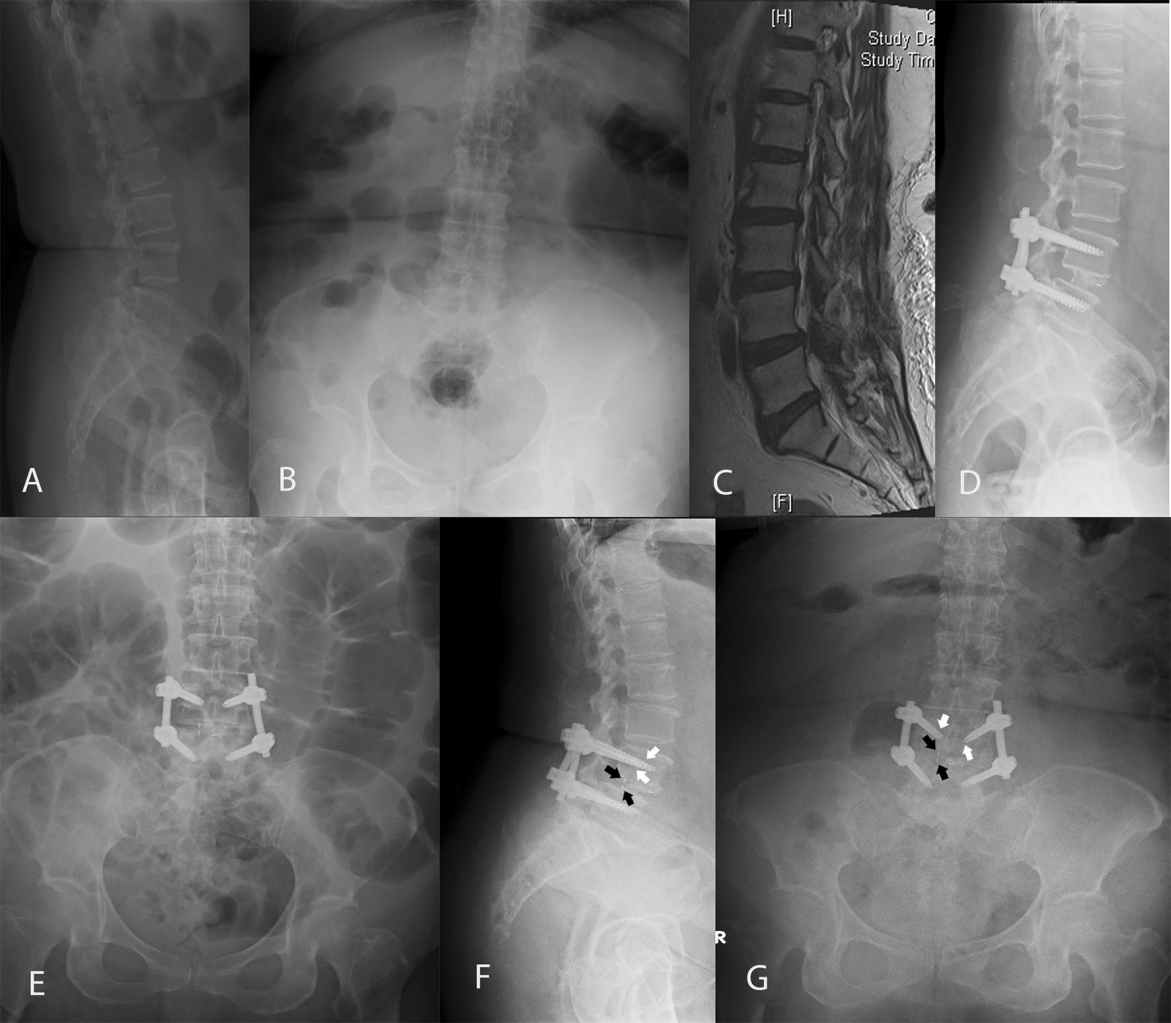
A patient was diagnosed preoperatively with degenerative spondylolisthesis and lumbar spinal stenosis at L4-5 level, and underwent minimally invasive transforaminal lumbar interbody fusion (MIS-TLIF). Radiographic images (A,B) and MRI (C) were taken preoperatively. The immediate post-operative lumbar spine lateral view (D) and lumbar antero-posterior view (E) are shown. Cage subsidence (black arrow) and screw halo sign (white arrow) were seen on the last follow-up images (F,G).
